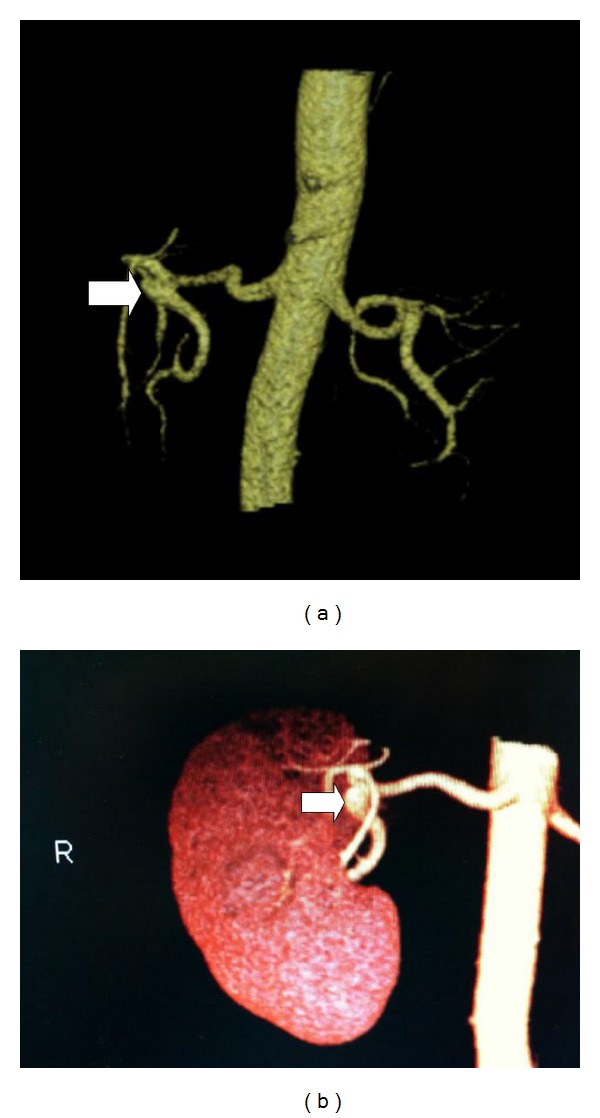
CT angiogram with 3D reconstruction shows a saccular aneurysm arising from the proximal right lower pole renal artery at the bifurcation of the main renal artery (*arrow*).
